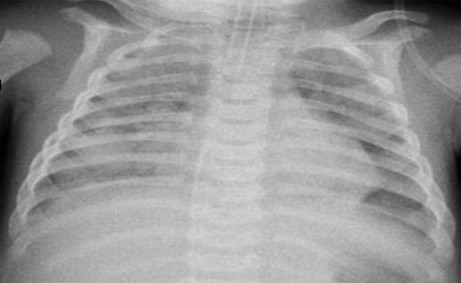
Chest X-ray of case 1.
